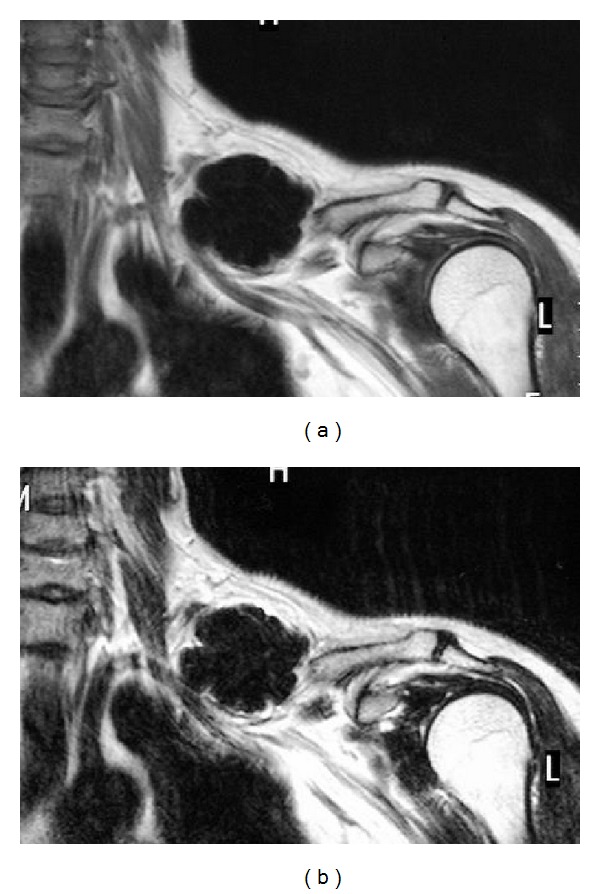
Magnetic resonance imaging reveals that the lesion has the same density as cortical bone. (a) A coronal T1-weighted image shows that the lesion has low signal intensity located in the midportion of the clavicle. (b) This coronal T2-weighted image shows the lesion with low signal intensity.
